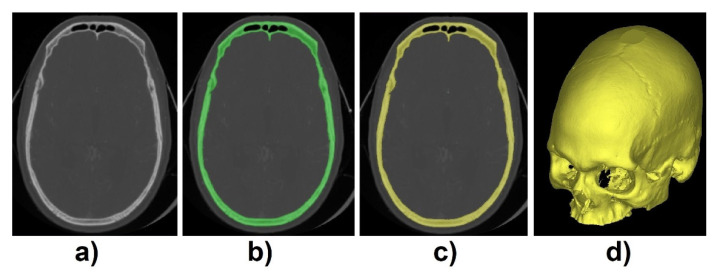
(a) Computed tomography (CT) scan, (b) thresholding applied, (c) region growing, and (d) 3D mesh model.
